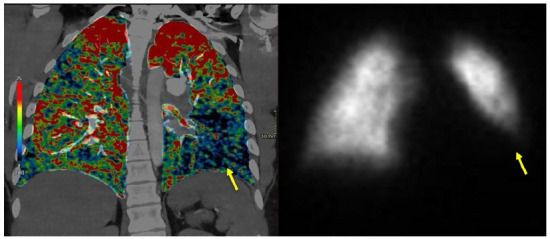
Dual-energy CT of the chest and lung perfusion scintigraphy. Dual-energy CT iodine map and lung perfusion scintigraphy used to investigate pulmonary hypertension. In this case, the two diagnostic methods agree and show hypoperfusion in the left lower lobe (yellow arrow).
